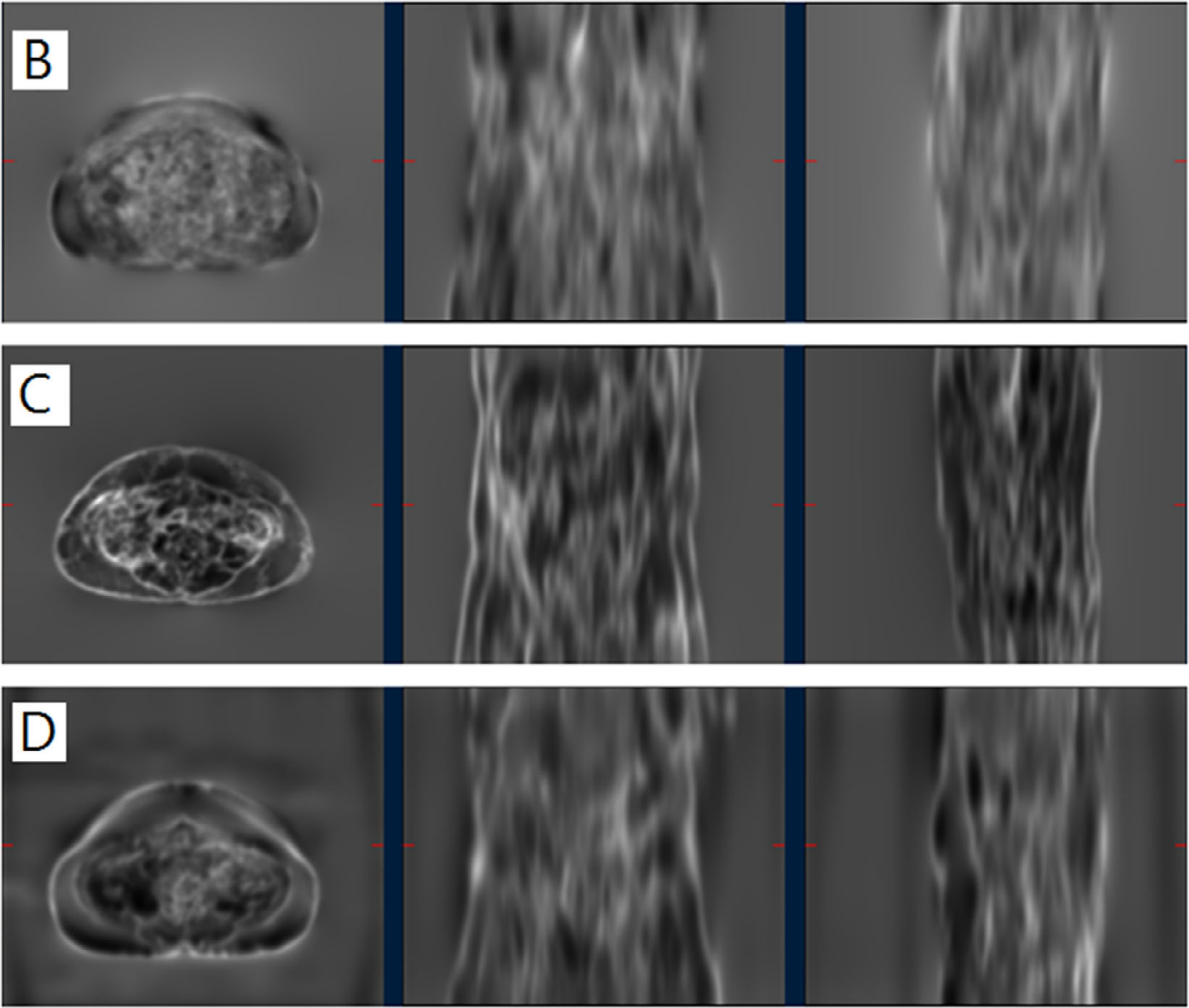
Jacobian determinant (JD) maps of the magnetic resonance (MR) opposed‐phase (OP) to computed tomography (CT) registration deformation fields of a typical subject, using the local phase difference (LPD)‐based method (B), the mutual information (MI)‐based method (C), or the proposed method (D). Note that the bright regions indicate local expansion, and the dark regions indicate shrinkage. These JD maps show the largest variation on the body edges and organ boundaries. Method B shows the largest deformation among the compared methods.
